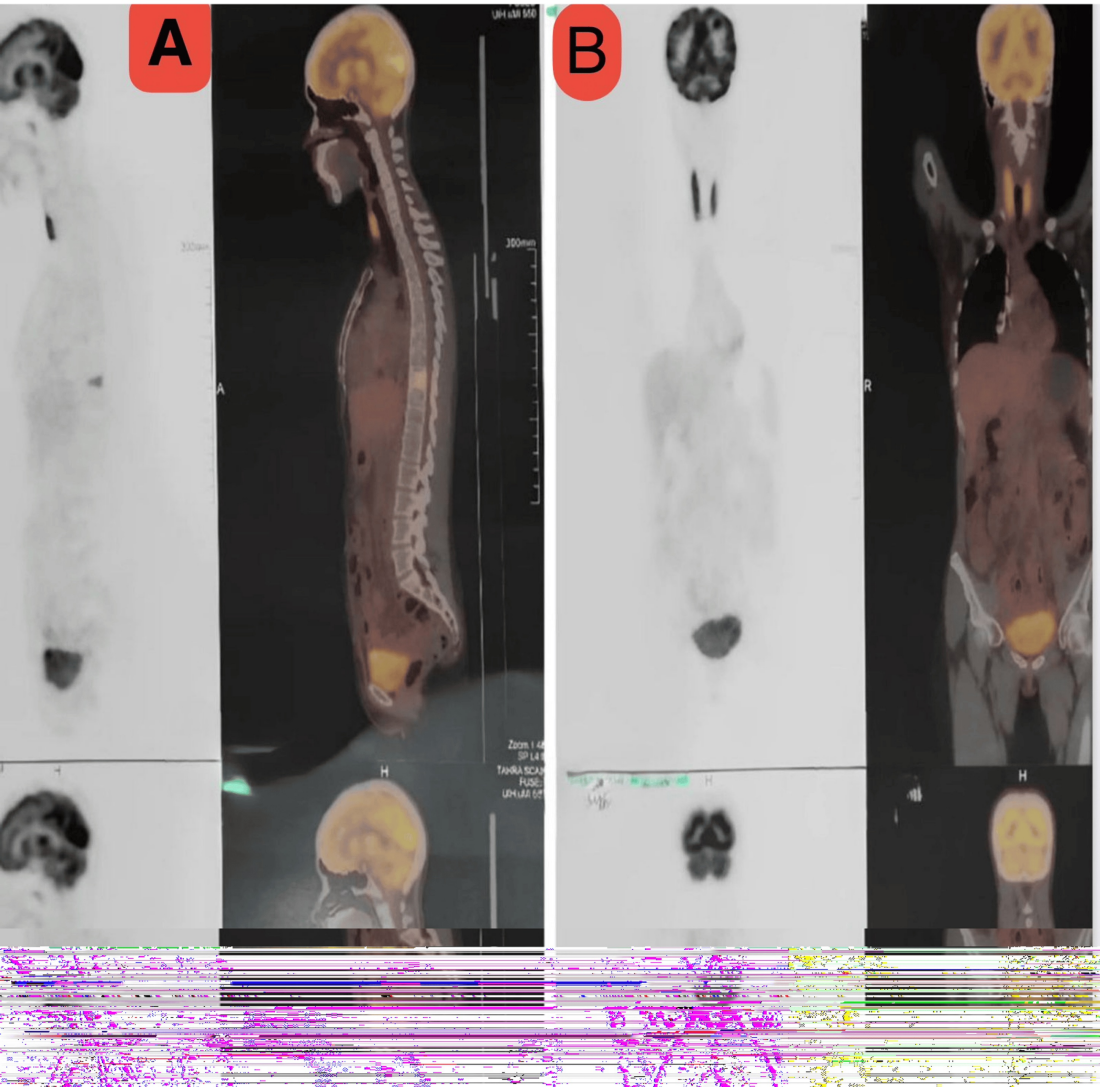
(A) Sagittal fused PET/CT images showing osseous metastases in the thoracic spine. (B) Coronal fused PET/CT images demonstrating peritoneal and osseous metastases PET/CT: positron-emission tomography/computed tomography
